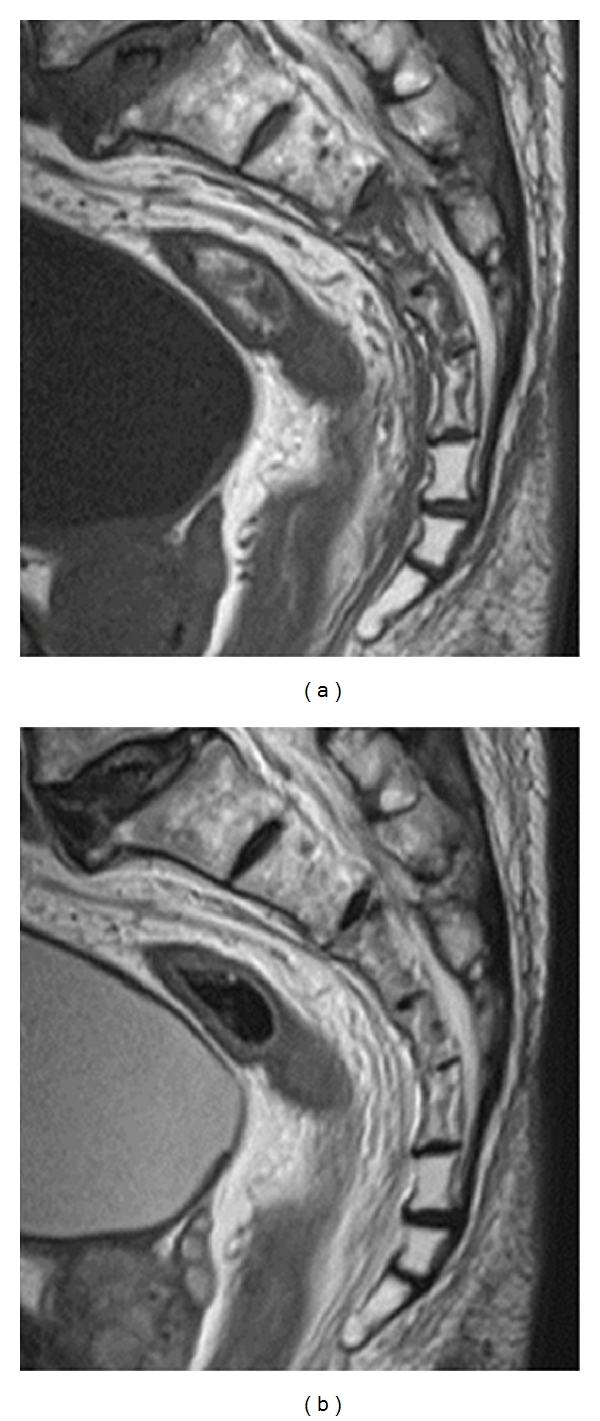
Preoperative magnetic resonance image. (a) Sagittal plane on a T1-weighted image and (b) sagittal plane on a T2-weighted image Third, fourth, and fifth sacral vertebral bodies showed low intensities on T1- and T2-weighted images.
